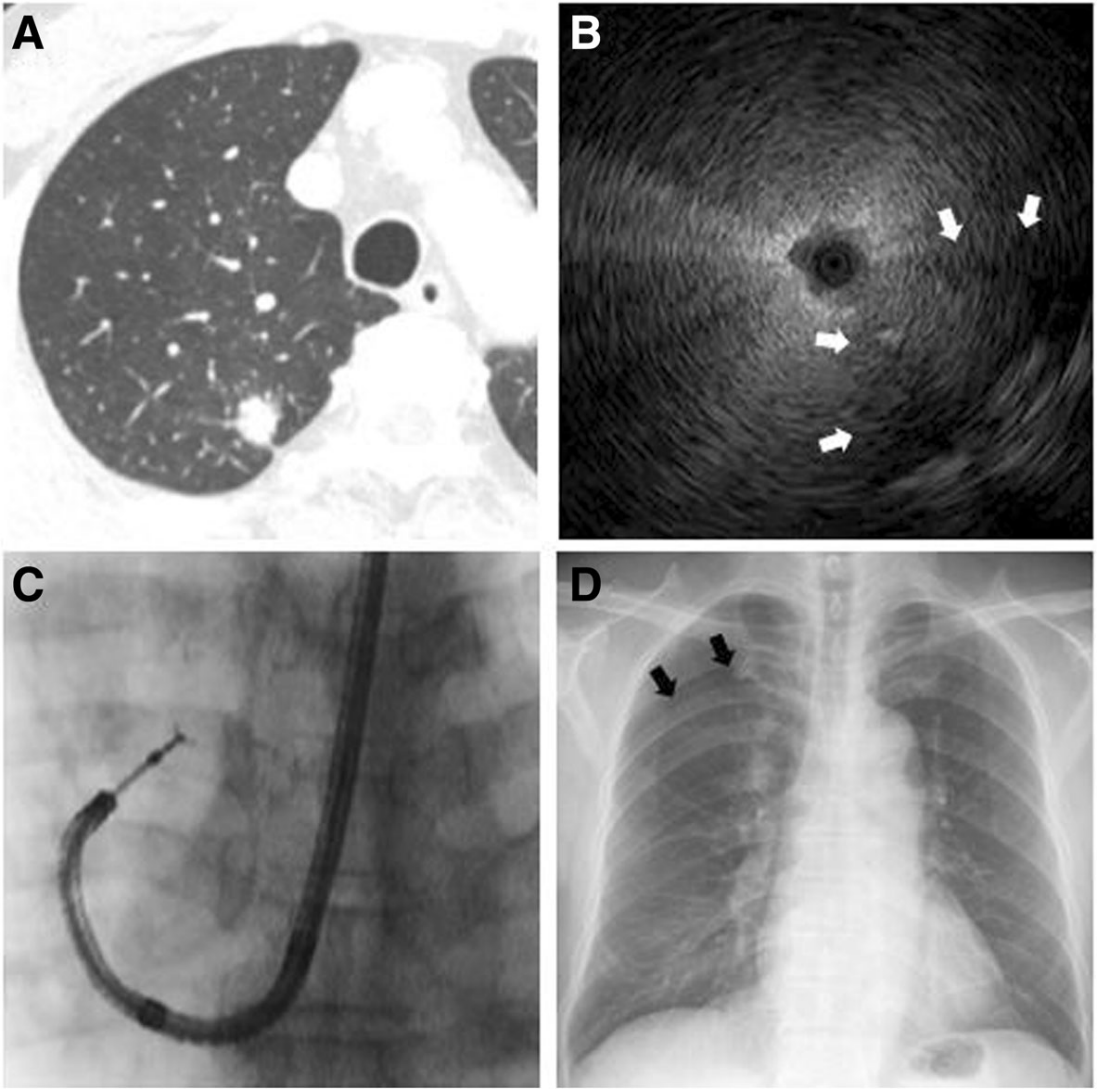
A patient who developed pneumothorax after the procedure.a A patient was admitted with a peripheral lung nodule measuring 15.1 mm at its greatest diameter and located in the right upper lobe, as seen on a chest computed tomography scan. b A radial probe endobronchial ultrasound (EBUS) image showed a hypoechoic area (white arrow) distinguishable from the normal aerated lung. c Under fluoroscopic guidance, transbronchial lung biopsy and brush cytology were performed via the guide sheath (GS). The diagnosis was adenocarcinoma. d Iatrogenic pneumothorax (black arrow) was identified on chest radiographs taken 4 h after EBUS-GS
